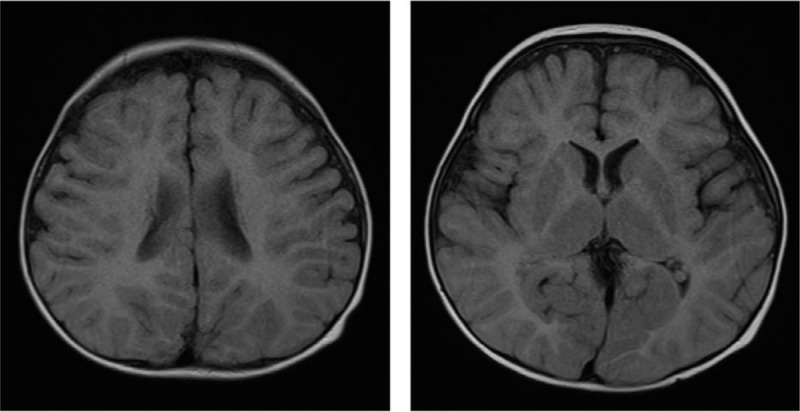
Brain MRI. MRI was performed when the patient was 11 months old. Brain MRI showed total demyelination in the bilateral white matter and dense bilateral thalami on T2W1.
